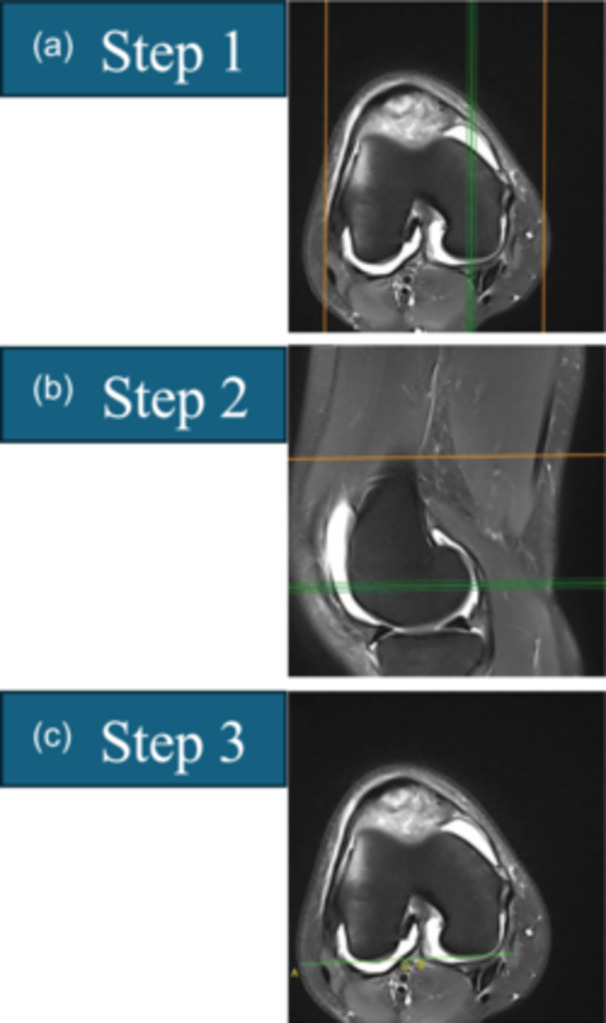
Identifying the posterior bicondylar line (PBCL). The PBCL is defined in three steps. (a) Step 1: Select the axial magnetic resonance imaging (MRI) slice that crosses the centre of the medial condyle. (b) Step 2: Select the sagittal MRI slice that crosses the most posterior point of the medial condyle. (c) Step 3: Return to the axial slice and draw the PBCL, which passes through the subchondral bone at the posterior aspect of the medial and lateral femoral condyles.
